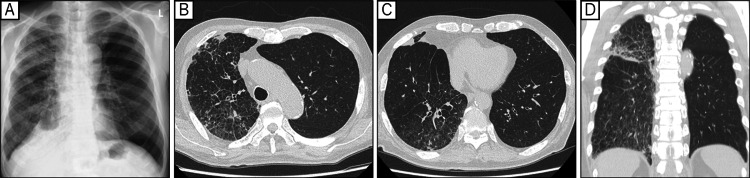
Post-treatment chest X-ray and CT images showing tumor regression (A-D) After starting dabrafenib+trametinib, the infiltrative shadow and ground-glass opacity due to lung cancer showed marked improvement on imaging.
